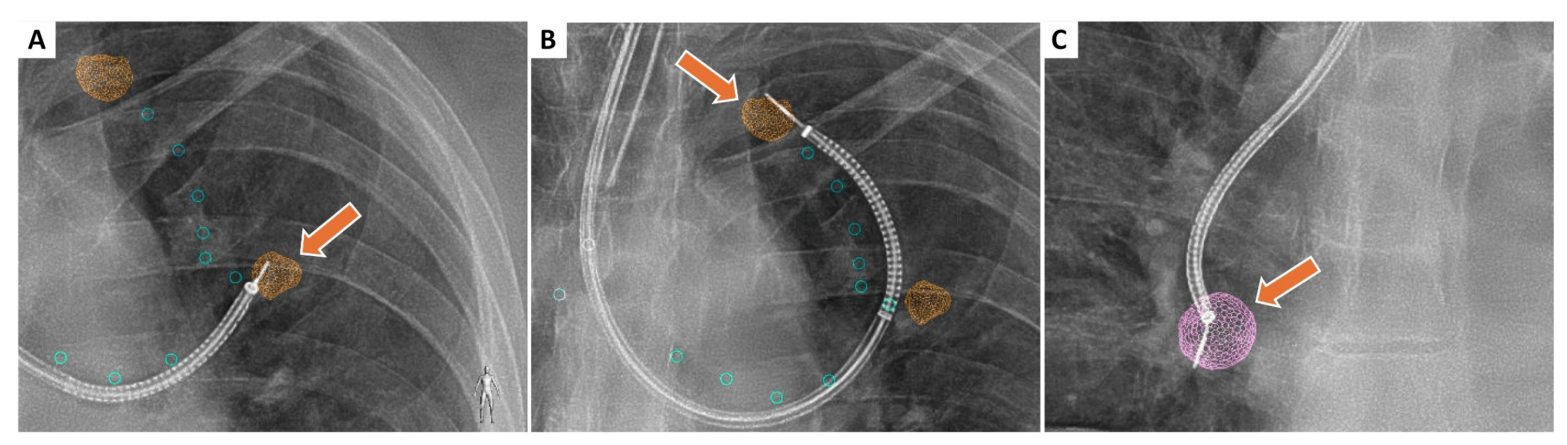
Fluoroscopic images obtained during robot-assisted bronchoscopy with cone-beam CT and augmented fluoroscopy. Orange arrows highlight the targeted pulmonary nodules in both lungs: (A) typical carcinoid, (B) well-differentiated adenocarcinoma, and (C) poorly differentiated adenocarcinoma. The orange and purple overlays represent the augmented fluoroscopy rendering of the nodule location in the left upper lobe and right lower lobe, and radial endobronchial ultrasound confirmation of lesion position prior to transbronchial biopsy.
